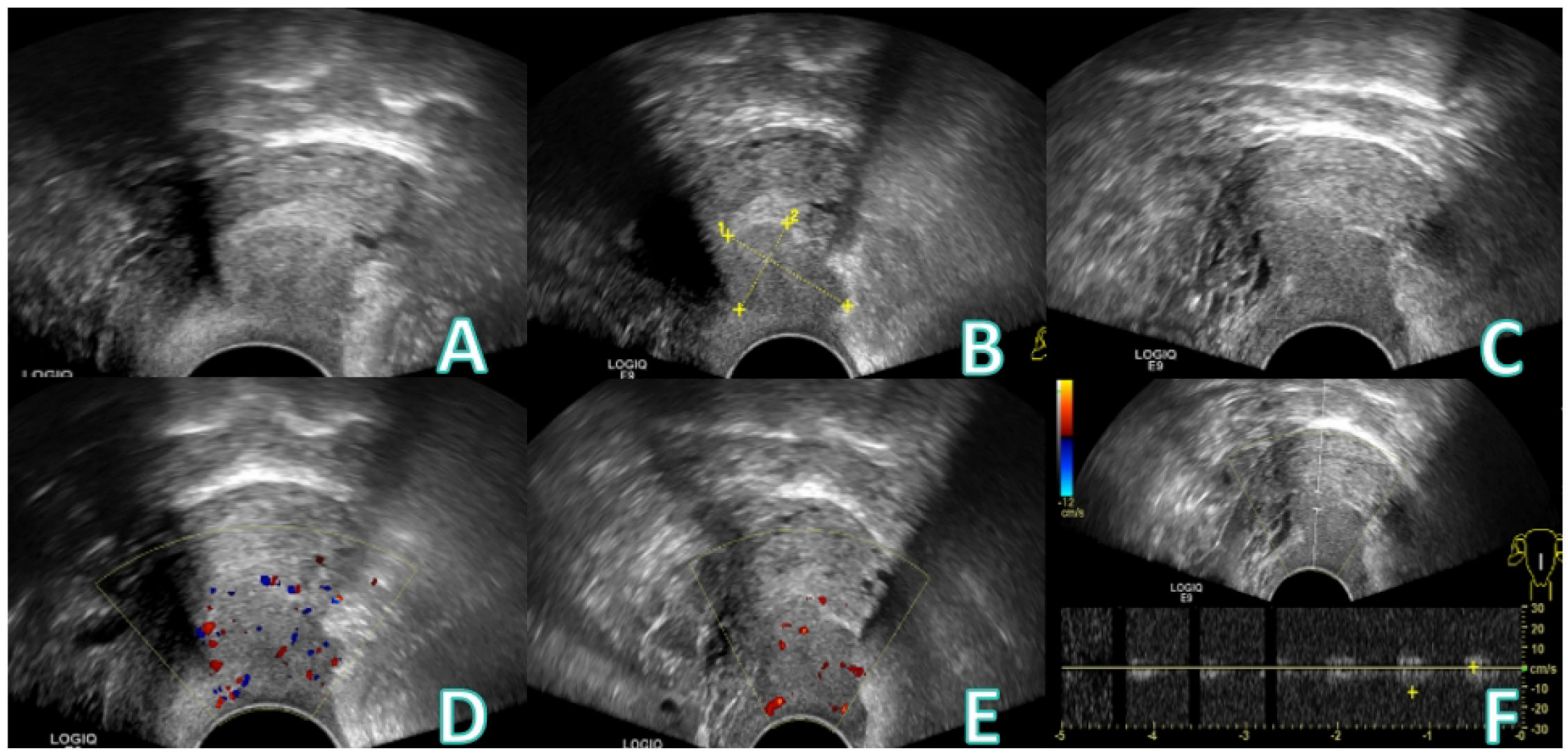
Conventional transvaginal ultrasound images: (A-C) A hypoechoic nodule, approximately 2.5×1.8 cm in size, is seen posterior to the uterus and poorly demarcated from it. (D) A few punctate blood flow signals can be seen in color Doppler. (E) Punctate blood flow signals are visible on spectral Doppler. (F) Arterial blood flow signal was detected.
